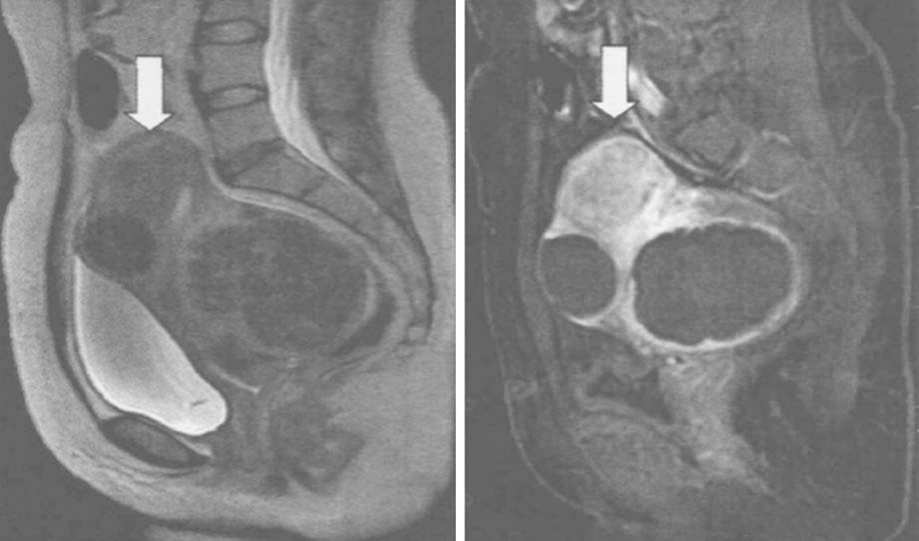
MRI images for UFs with different locations. Left: Before HIFUA; right: after HIFUA.
